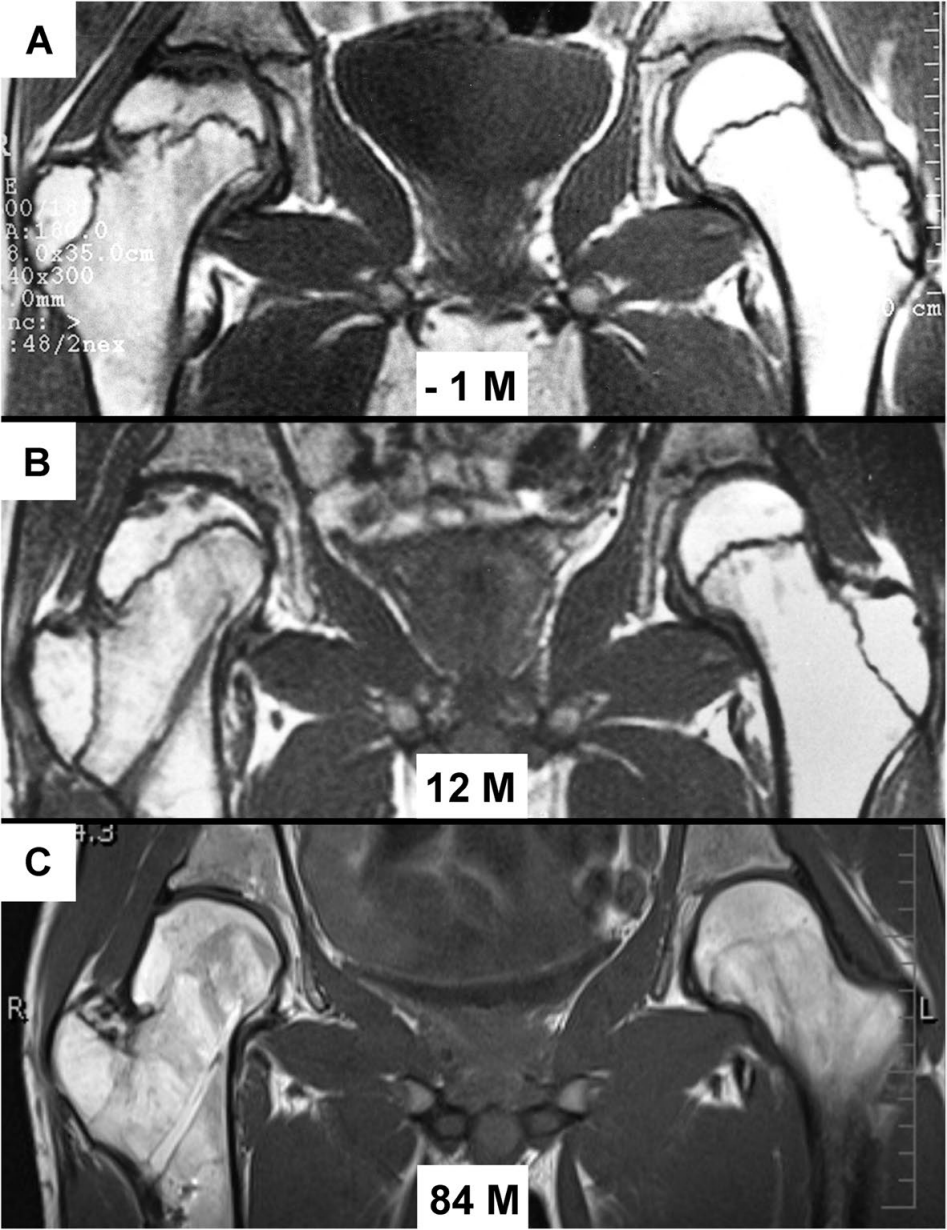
Remodeling of proximal femur in patient N1 on MRI. a hip joint one month before surgery; b situation 12 months after surgery, with a clearly visible change in the shape of capital physis of injured femur; c situation 84 months after surgery
